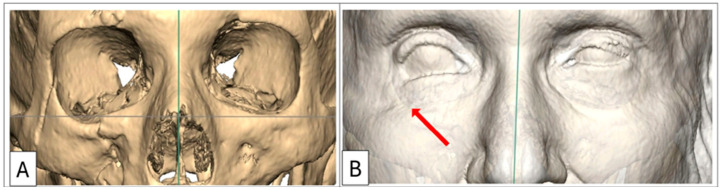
Case 1: Preoperative 3D-CT scan demonstrating lower eyelid retraction due to traumatic deformity (red arrow), requiring an infraorbital rim onlay implant and a lateral tarsal strip canthoplasty. Inferior displacement of the right lateral and infraorbital rim, (B) bone window, (A) soft-tissue window.
